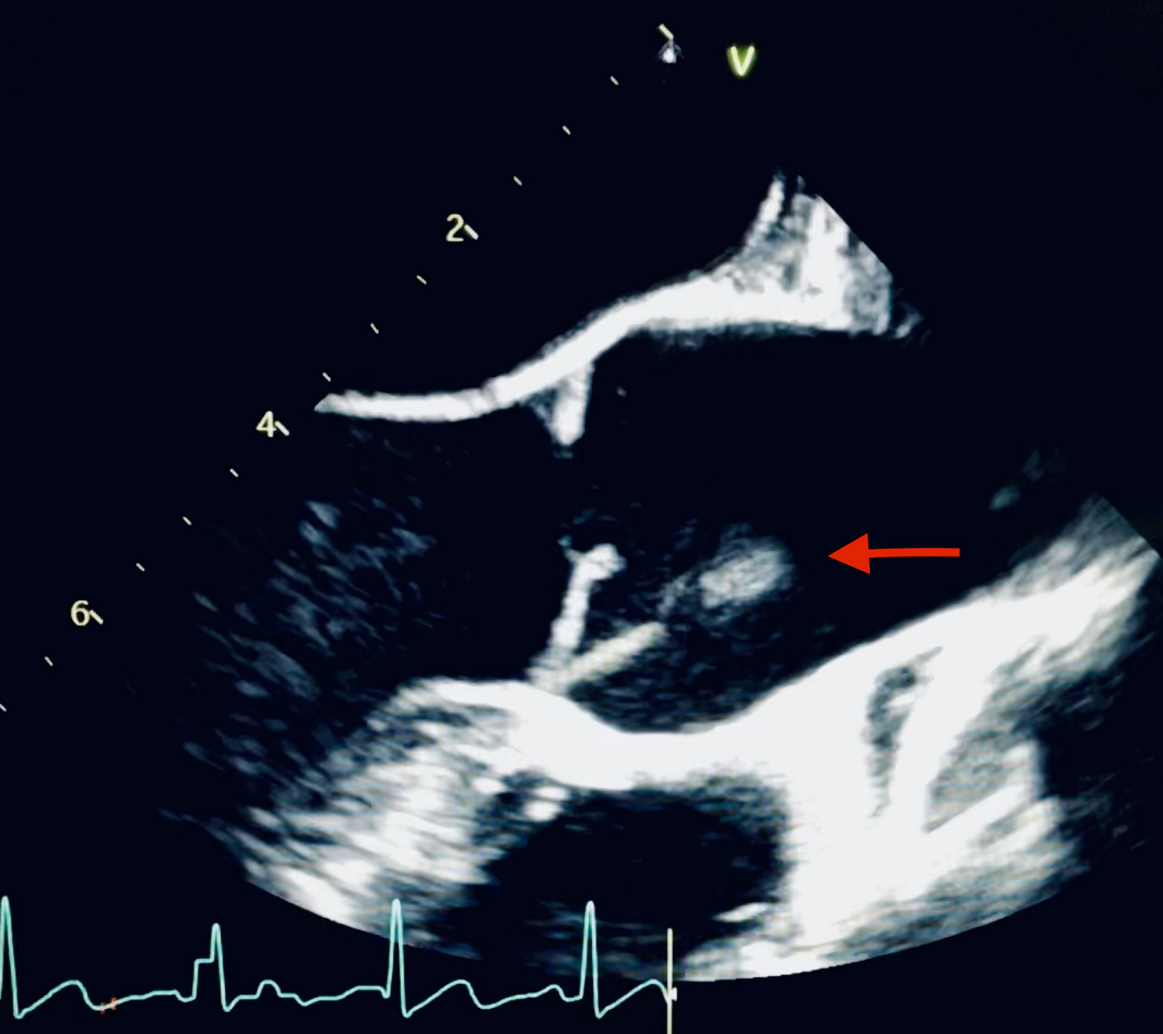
TEE showing an echodensity in the aortic root (red arrow). TEE, transesophageal echocardiogram
